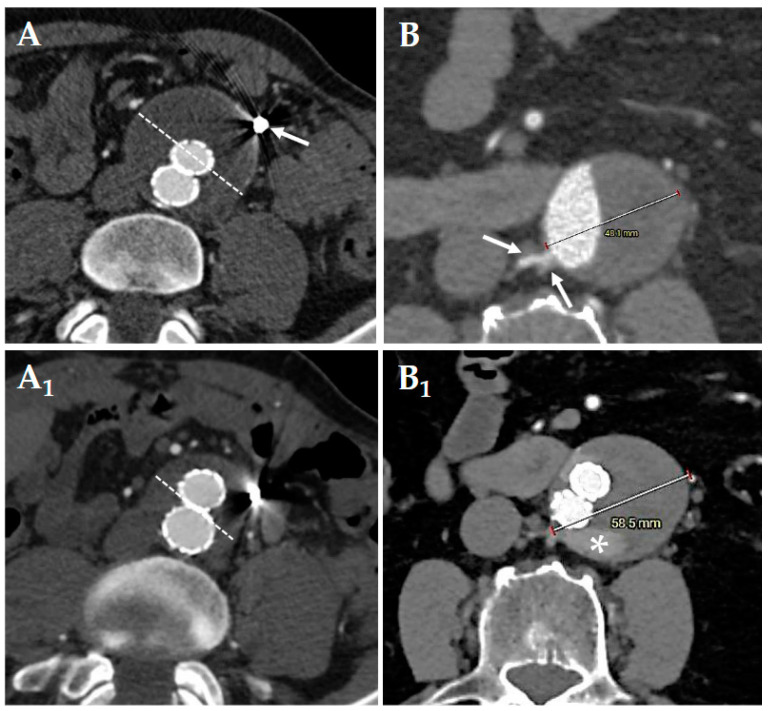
Preoperative (A) computed tomography–angiography of pre-emptive embolization of the inferior mesenteric artery ((A), white arrow). Postoperative (A_1_) follow-up shows a shrinkage of the aneurysmatic sac ((A,A_1_); sketched white lines). Preoperative (B) computed tomography–angiography of standard endovascular aortic repair without lumbar (white arrows) pre-emptive embolization. Postoperative (B_1_) follow-up at 2 months shows sac enlargement (48 mm-to-58 mm) due to the presence of a type 2 endoleak with the “nidus” (white asterisk) fed by the pair of lumbar arteries highlighted at the preoperative computed tomography–angiography.
